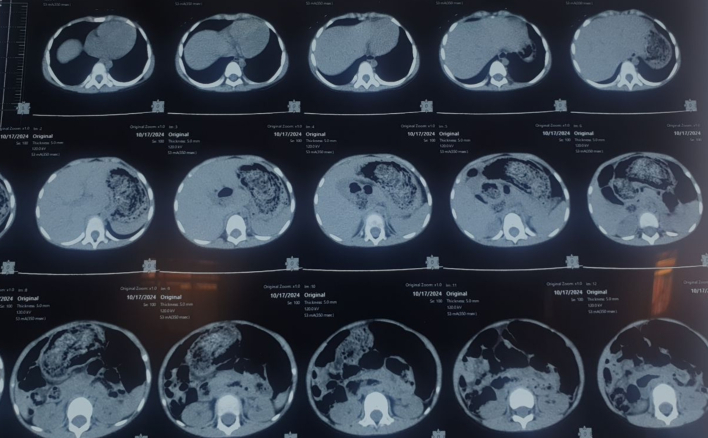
CT abdomen showing a moderately distended stomach with ill-defined heterogeneous content extending to the duodenum and suggesting Bezoars.
